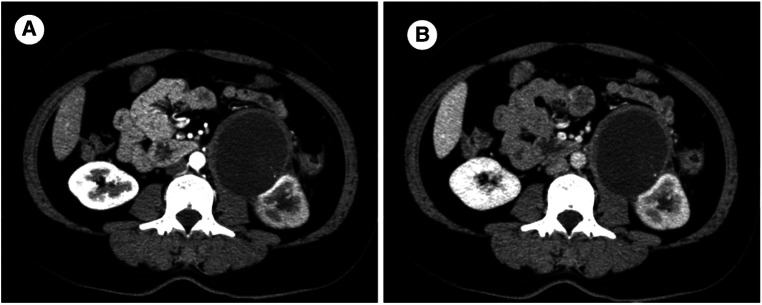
Enhanced computed tomography scan: A round low-denisty cystic mass located at the renal helium, measuring 7.4 × 7.4 cm in size with a width of 0.5 cm at the wall. (A) Arterial phase (B) Venous phase.
